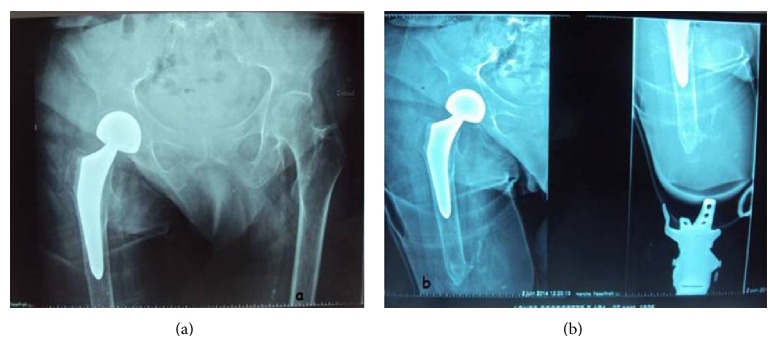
Pelvis and hip radiographies (an anteroposterior X-ray), at the follow-up of 24 months after the hemiarthroplasty.
